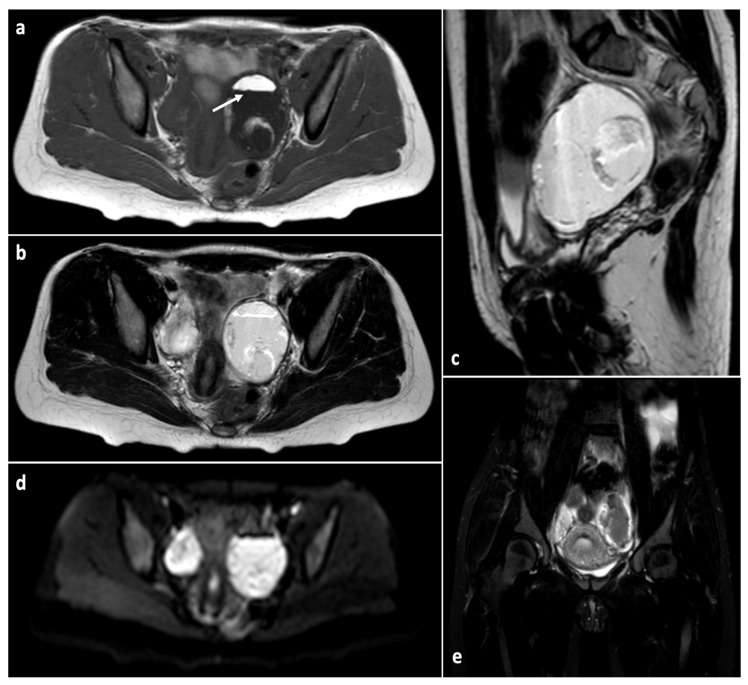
Magnetic resonance imaging sequences consistent with mature teratoma of the left ovary in an adolescent patient. (a) Axial T1-weighted image demonstrating the fat–fluid level (arrow) with hyperintense fat/sebaceous material on the non-dependent component of the mass; (b,c) axial and sagittal T2-weighted images, respectively, showing high signal of the cyst contents; (d) axial diffusion-weighted image revealing high signal of the dependent portion of the lesion; (e) coronal T2-weighted fat-suppressed image exhibiting signal drop within the mass.
